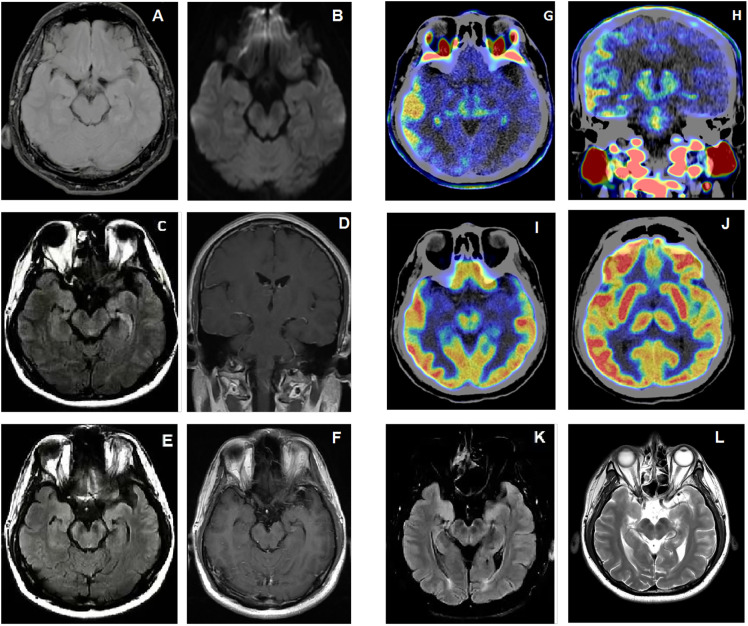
(A, B) On the first admission, FLAIR and DWI sequences showed normal (C, D) On the second admission, FLAIR sequences and Contrast-Enhanced T1-weighted sequences of the patient with a series new symptoms onset revealed swelling temporal lobe and enhanced leptomeningeal of right cerebral hemisphere. (E, F) After the treatment of IVMP and IVIG, FLAIR sequences showed increased intensity in the bilateral hippocampi and mesencephalon, and contrast-enhanced T1-weighted sequences showed patchy linear enhancement in the meninges. (G, H) 18F-DPA714 PET/CT showed higher distribution of 18F-DPA714 in right frontal temporoparietal lobe. (I, J) 18F-FDG PET/CT showed hyper metabolism of fluorodeoxyglucose in right frontal temporoparietal lobe. (K, L) After 1 year follow up, FLAIR and T2-weighted sequences showed reduced lesions in the bilateral hippocampi and mesencephalon compared to before.
